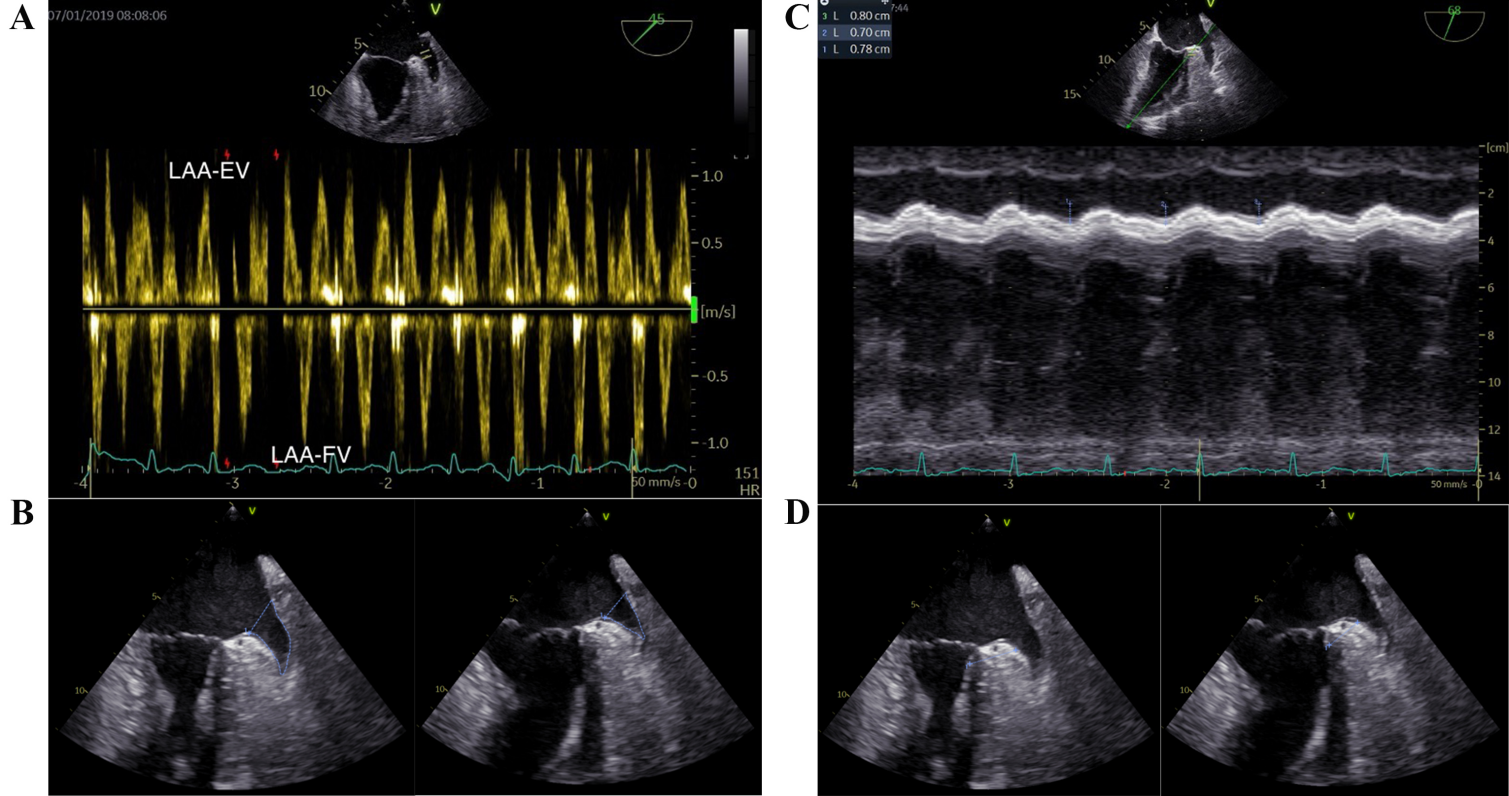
Key transesophageal echocardiographic parameters in the LAA
